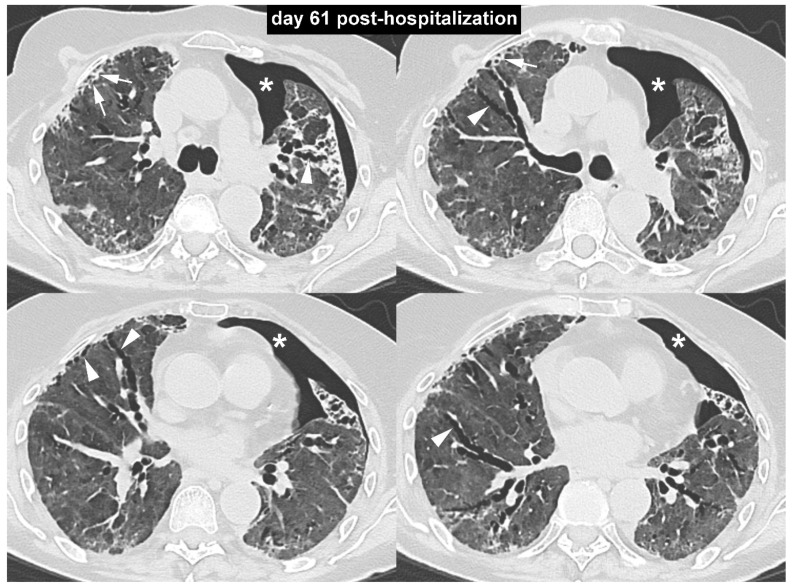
The subsequent bedside radiographic follow-up showed a trend toward gradual reduction in pulmonary opacities. On day 61 post-hospitalization, owing to persistent breathlessness and the need for high-flow oxygen therapy, an unenhanced high-resolution chest CT scan was performed. The high-resolution CT images with lung window setting (Figure 3) demonstrated the persistence of a diffuse increase in lung density with ground-glass appearance, associated with peripheral irregular reticular opacities (arrows) and several traction bronchiectasis (arrowheads). These findings were consistent with the diagnosis of pulmonary fibrosis. In addition, an unexpected left-sided spontaneous pneumothorax was noted (asterisks).
